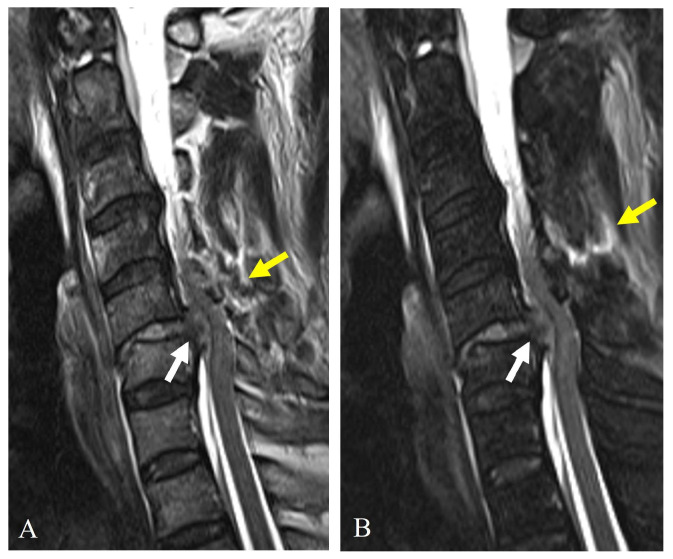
Magnetic resonance imaging (A,B) reveals traumatic disc rupture (white arrows) compressing the spinal cord and posterior ligament complex injury (yellow arrows) at C5–C6.
